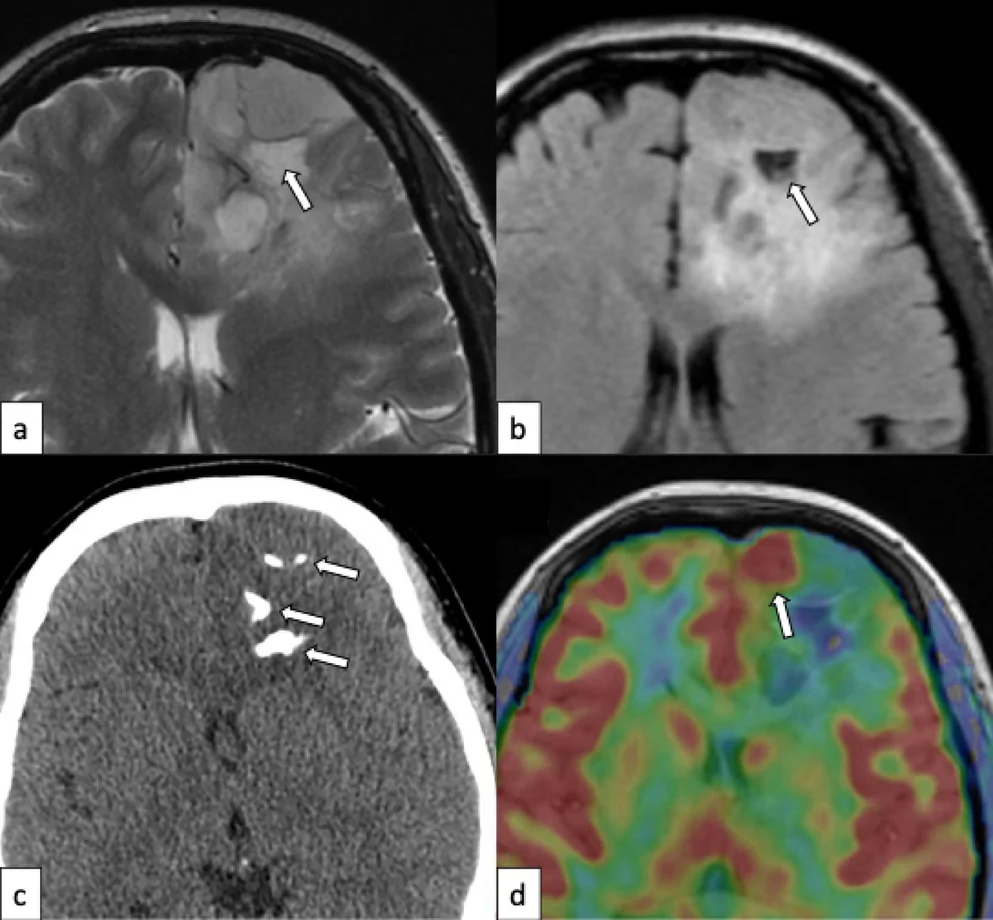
Oligodendroglioma, IDH-mutant and 1p/19q-codeleted, CNS WHO grade 3 in a 43-year-old woman. The tumor mass located in the cortico-subcortical region in the left frontal lobe shows hyperintensity on T2-weighted image (a) and on fluid-attenuated inversion recovery (FLAIR) image (b). Subcortical FLAIR signal drop is positive in this case (a, b, arrows). Coarse calcification is observed within the tumor mass on non-enhanced computed tomography (c, arrows). Cortical high-flow sign is observed on arterial spin labeling (d (fusion image), arrow)
